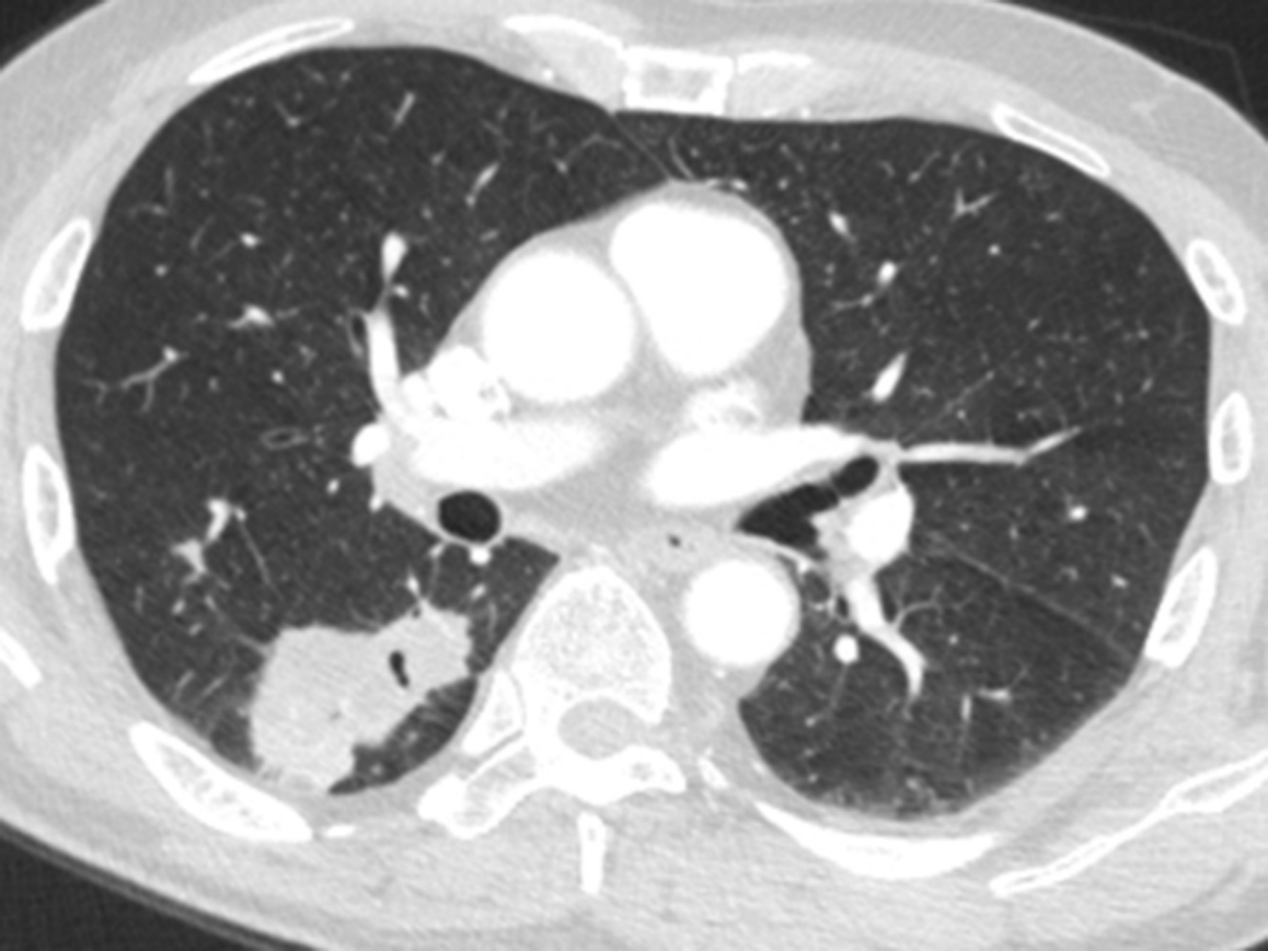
Case 3: Axial CT image of the thorax in lung window shows a mass with irregular margins in the right lower lobe.
